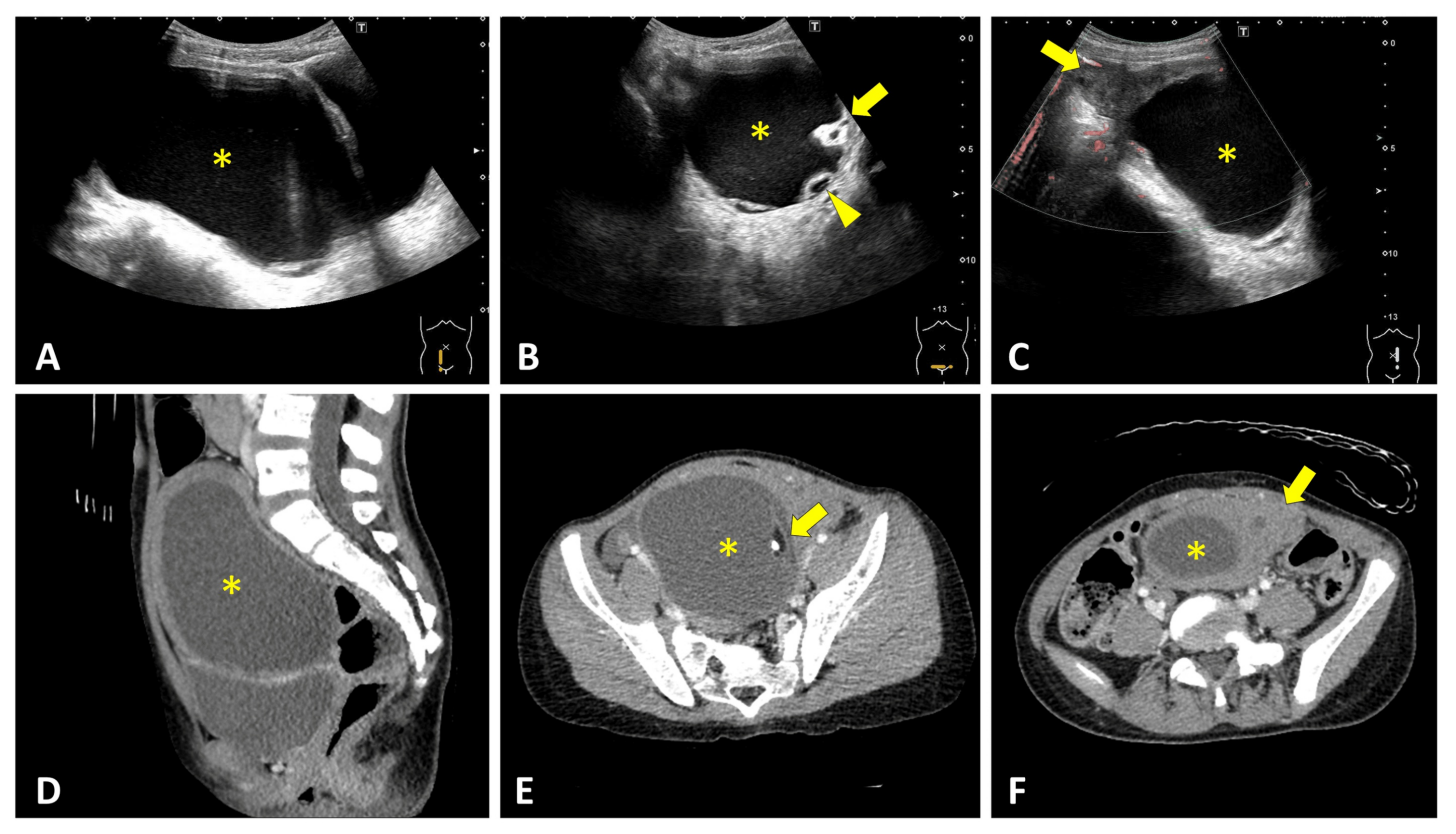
Double-Bladder Sign on RADUS With Corresponding CT Findings Longitudinal RADUS (A) demonstrated a cystic mass (*) producing the double-bladder sign. The transverse view (B) depicted a daughter cyst sign (arrowhead) and calcification (arrow). Color Doppler ultrasound showed partial flow within the enlarged left ovarian lesion (C, arrow). Sagittal CT (D) demonstrated a large cystic mass (*) consistent with the double-bladder sign observed on ultrasound. Axial CT revealed calcification and fat (E, arrow) within the cystic mass (*) and an enlarged left ovary with follicles (F, arrow). RADUS: radiology-performed ultrasound
